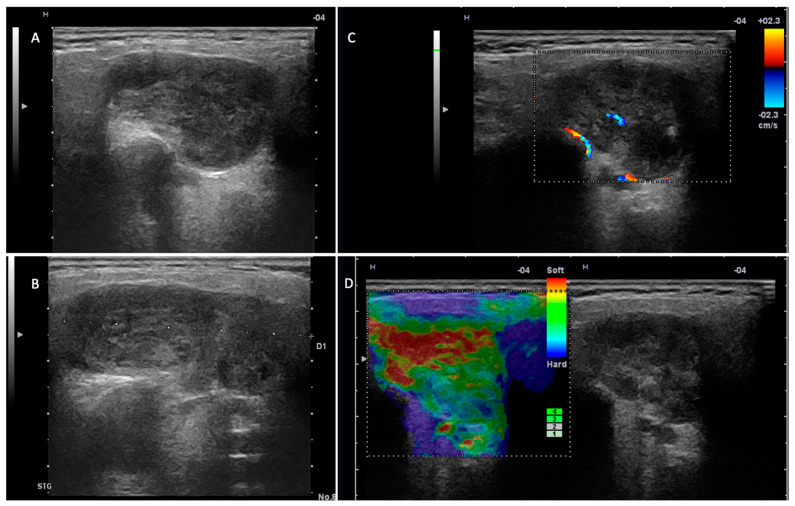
Pleomorphic adenoma—left parotid gland. (A,B) Two-dimensional examination revealing a hypoechoic nodule, with an irregular contour, slightly lobulated, and well demarcated from the adjacent parotid parenchyma. (C) The color Doppler examination reveals a reduced intralesional vascular signal. (D) Elastography examination with a predominantly soft intratumoral appearance.
